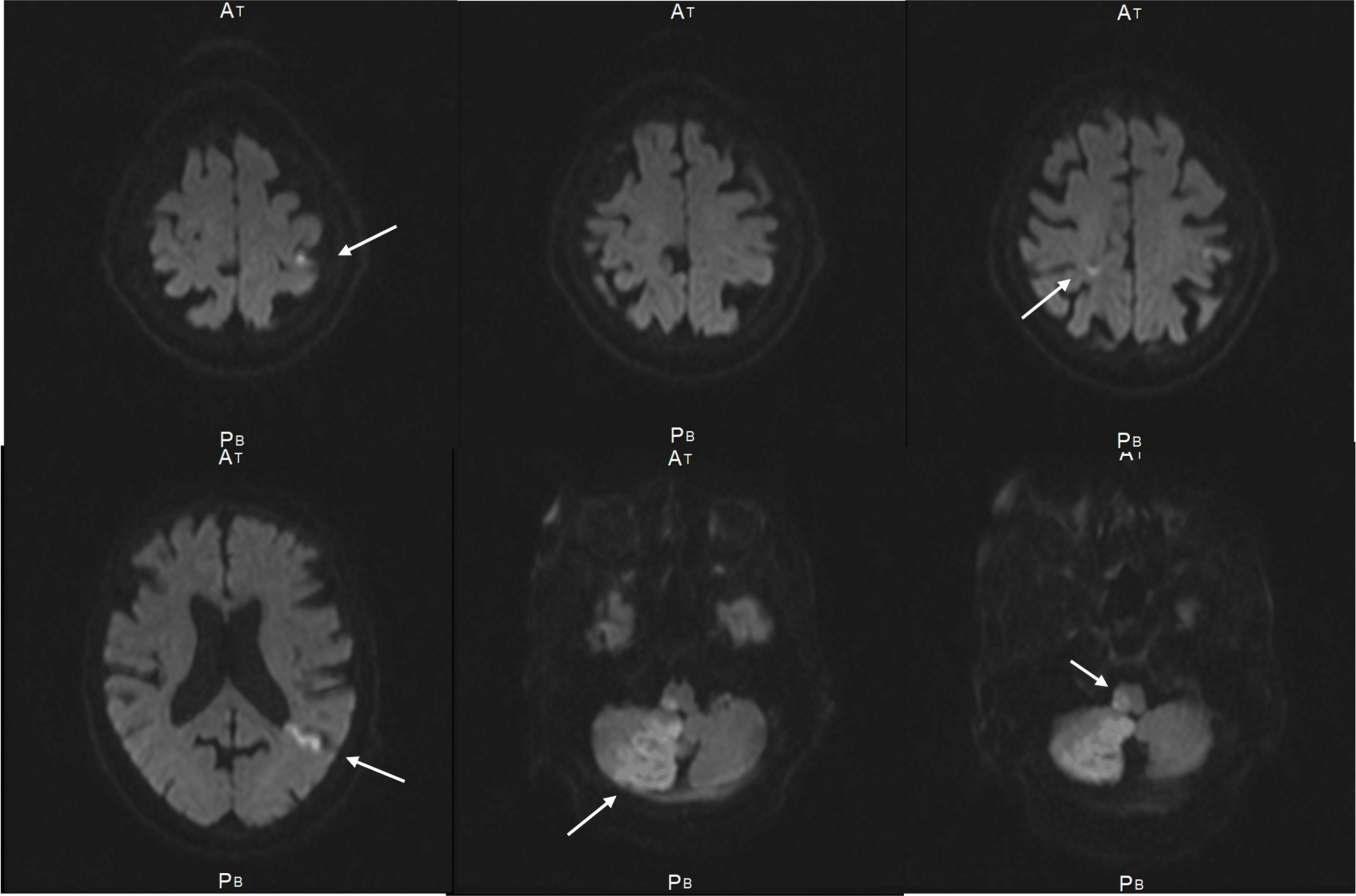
Multiple fresh brain infarctions (Case 3). MRI with diffusion-weighted imaging at initial consultation showed multiple acute infarctions in the right cerebellum, right brainstem, and bilateral temporal/parietal lobes (arrows), contributing to the patient's impaired consciousness. MRI: magnetic resonance imaging.
